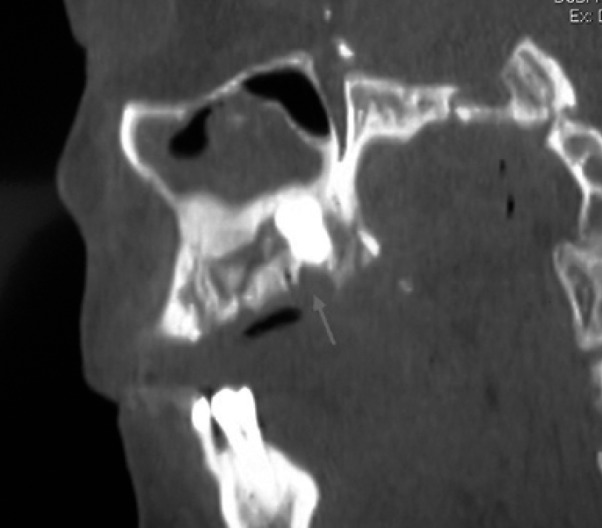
Sagital CT image demonstrating bone sequestrum and third molar inclusion (red arrow).
